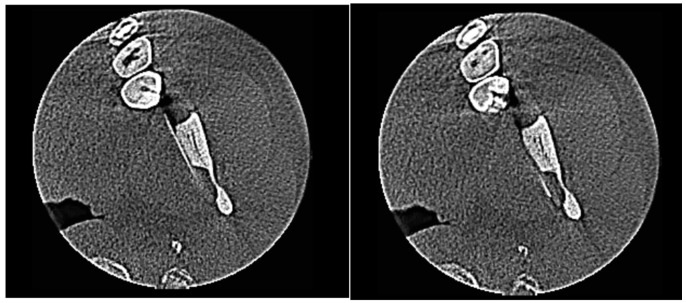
Axial CBCT slices confirming the retained needle fragment.
